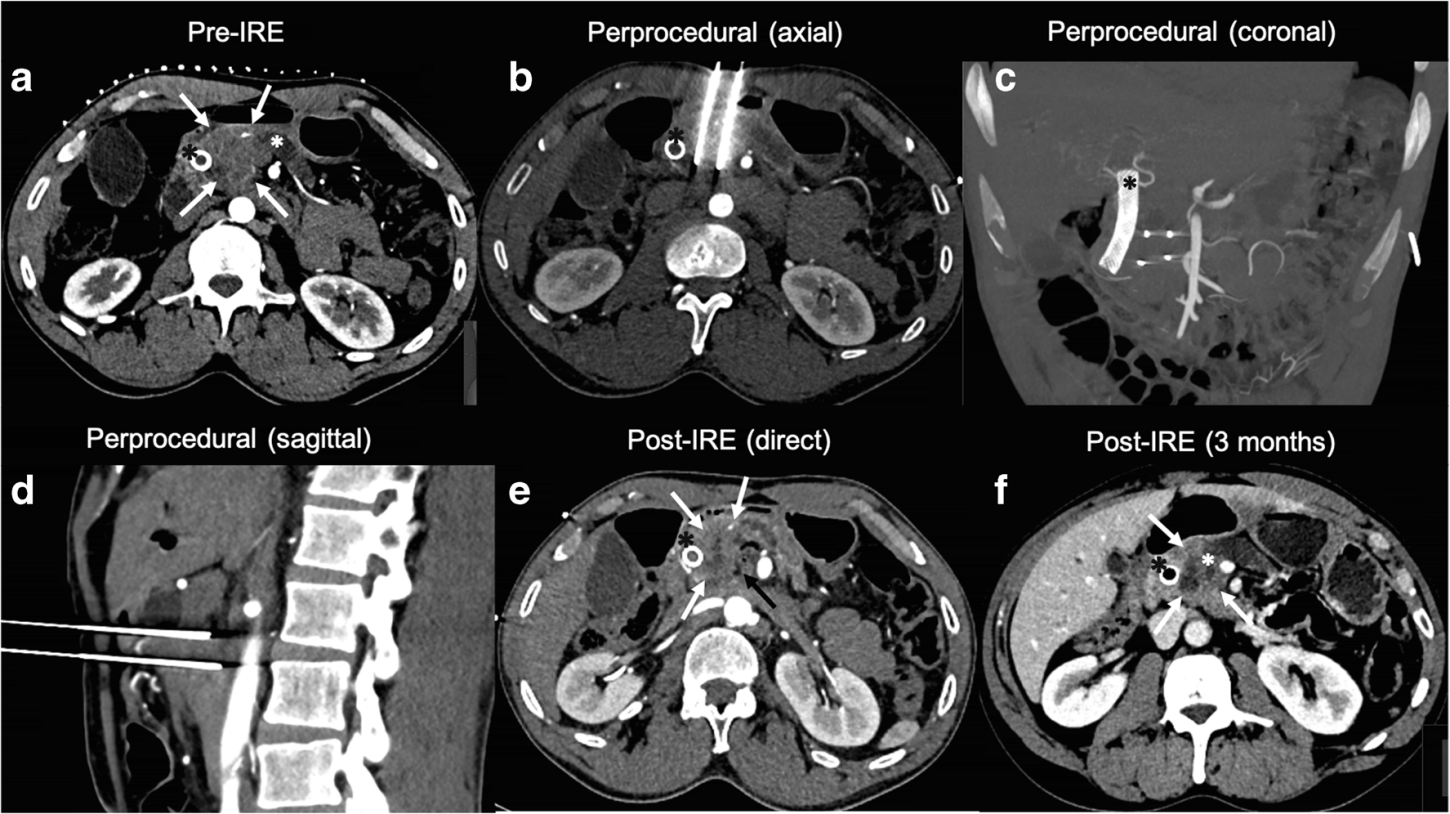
CT-guided percutaneous irreversible electroporation (IRE) for locally advanced pancreatic cancer (LAPC). Sixty-two-year-old male with LAPC on the basis of involvement of the superior mesenteric artery (0–90°, although complete encasement (360°) of the first jejunal branch), involvement of the aorta (0–90°), and involvement of the superior mesenteric vein/portal vein (0–90°). A biliary stent (black asterisks in a, b, c, e, f) was placed prior to IRE using endoscopic retrograde cholangiopancreatography (ERCP). a Perprocedural contrast enhanced (ce)-CT of the LAPC in the head of the pancreas (white arrows) and biliary stent (black asterisk) prior to IRE treatment. The white asterisk shows significant dilation of the pancreatic duct. b Perprocedural axial view of 2 of the 4 needle electrodes in situ. c Perprocedural coronal view of all 4 needle electrodes in situ. The needles were successfully placed, bypassing all major blood vessels. d Sagittal view of 2 of the 4 needle electrodes in situ. e ce-CT immediately after IRE. The white arrows delineate the ablation zone, wherein formation of gas pockets is clearly visible (black arrow). The gas pockets maybe the result of water electrolysis and/or vaporization. f ce-CT 3 months post-IRE demonstrates a hypointense ablation zone (white arrows). The portal vein is open; dilation of the pancreatic duct (white asterisk) remains unchanged. No evidence of local recurrence or distant metastases
